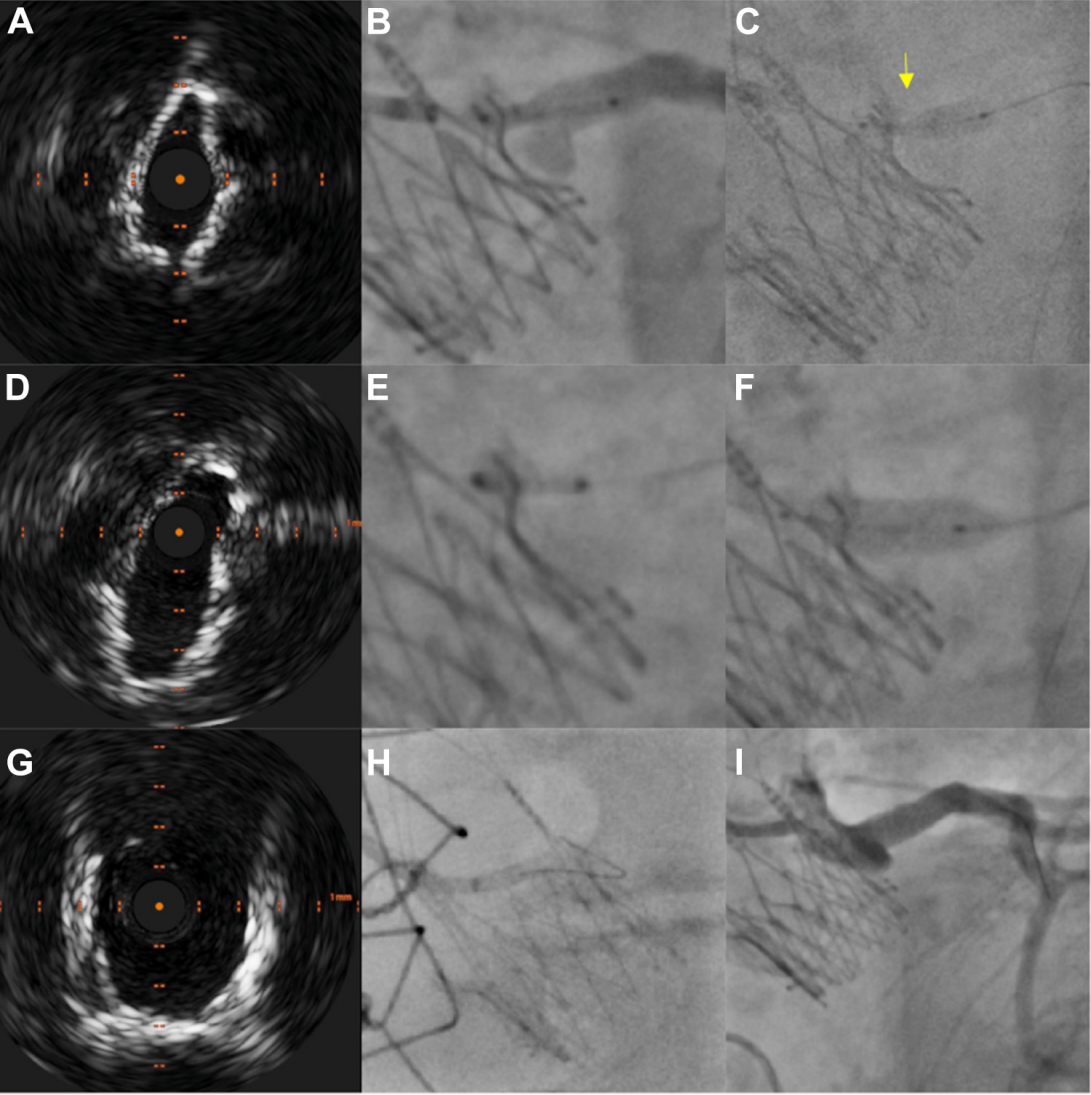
Chimney Stenting and Intravascular Ultrasound Relevant left main ostial obstruction on intravascular ultrasound (IVUS) (A). Positioning of the first coronary stent within the upper crown of the ACURATE neo2 prosthesis (B). Distinct narrowing during inflation of the coronary stent (yellow arrow, C). Owing to a slit-like narrowing of the first deployed coronary stent (D), a second coronary stent was implanted (E). After proximal optimization (F) there was a good final result with widely flared ostial stent portion (G). After inadvertent withdrawal of the guiding catheter, successful selective re-engagement and insertion of a coronary wire for final IVUS assessment (H) with patent left coronary artery and TIMI flow grade III (I).
